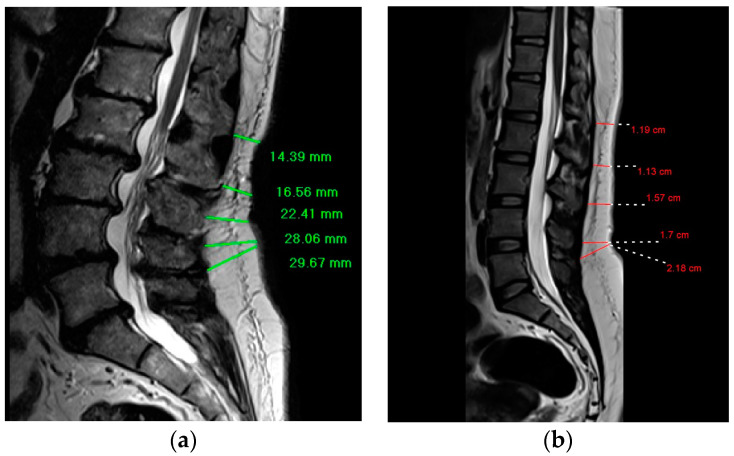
Demonstrates the way of the DSFT measurements for participants with degenerative disc disease and participants without the disease; (a) Mid-sagittal T2-weighted magnetic resonance image shows the measurement of the DSFT in a patient with worse degenerative disc disease at L1–L2 and L2–L3; (b) Mid-sagittal T2-weighted magnetic resonance image shows the measurement of the DSFT in a patient without the disease.
